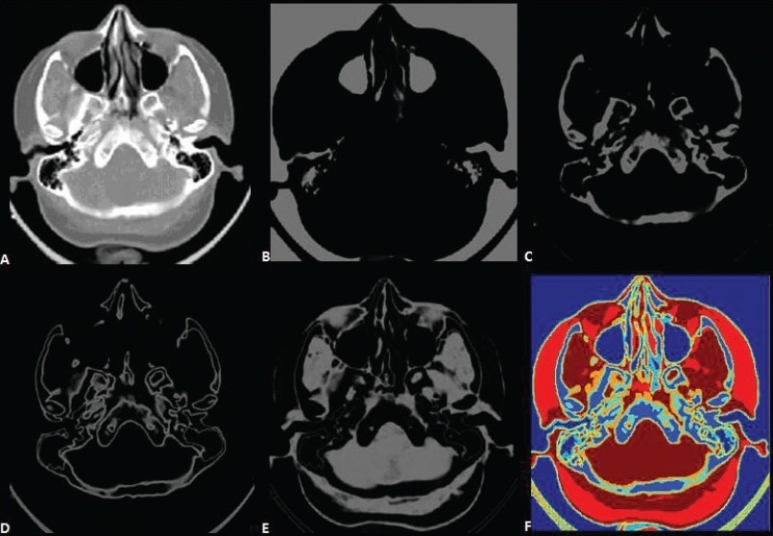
Individual Segments of Brain CT Image (A) Original (B-E) Individual segments (F) Segmented image in Pseudo Color
